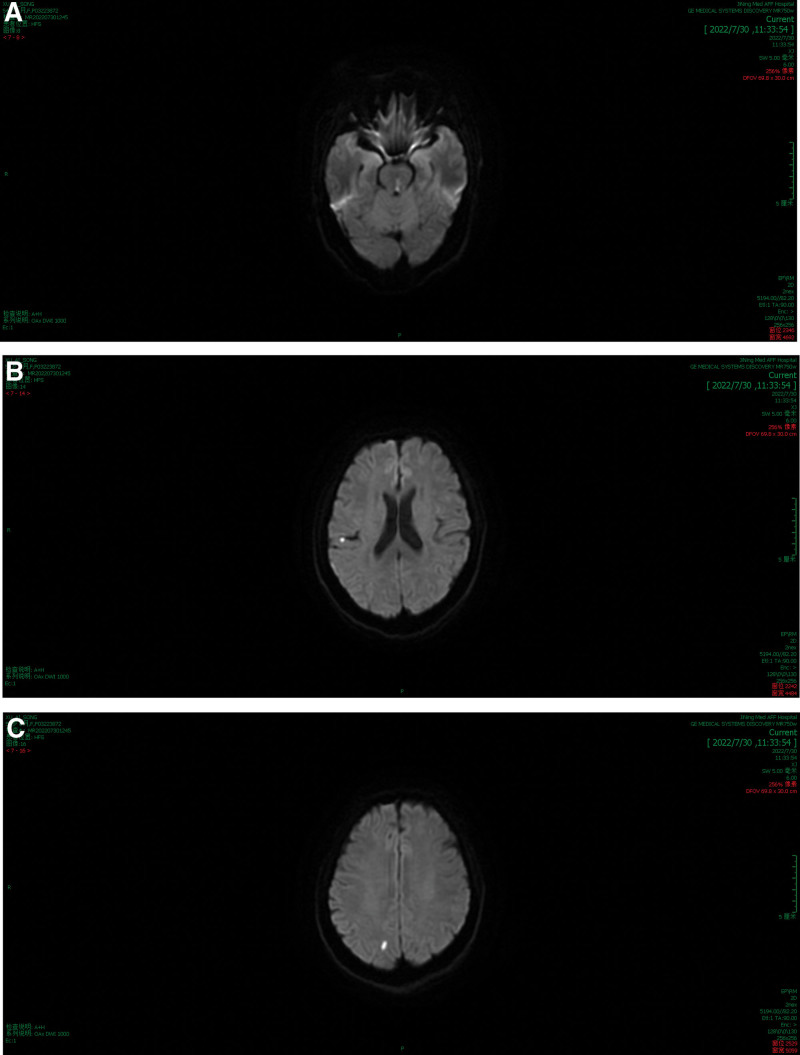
Cranial MRI showing high signal on DWI images. (A) Pons. (B) Parietal lobe. (C) Occipital lobe. DWI = diffusion-weighted imaging, MRI = magnetic resonance imaging.
